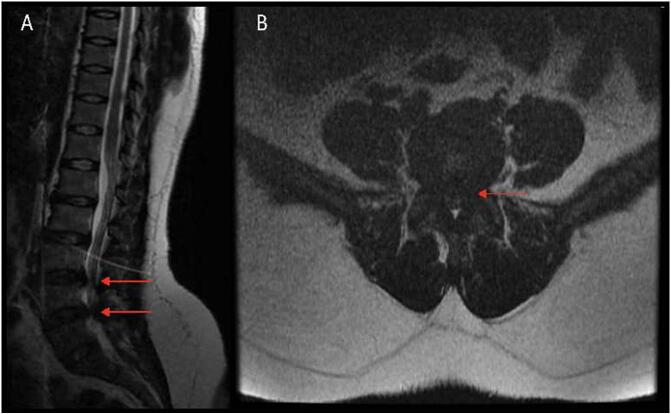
Patient 1: Preoperative MRI. (A) Sagittal T2-weighted MRI showing central stenosis at L3–4 and L4–5, with the most stenotic level being the latter. (B) Axial T2-weighted image showing significant central and lateral recess stenosis, worst at L4–5.
